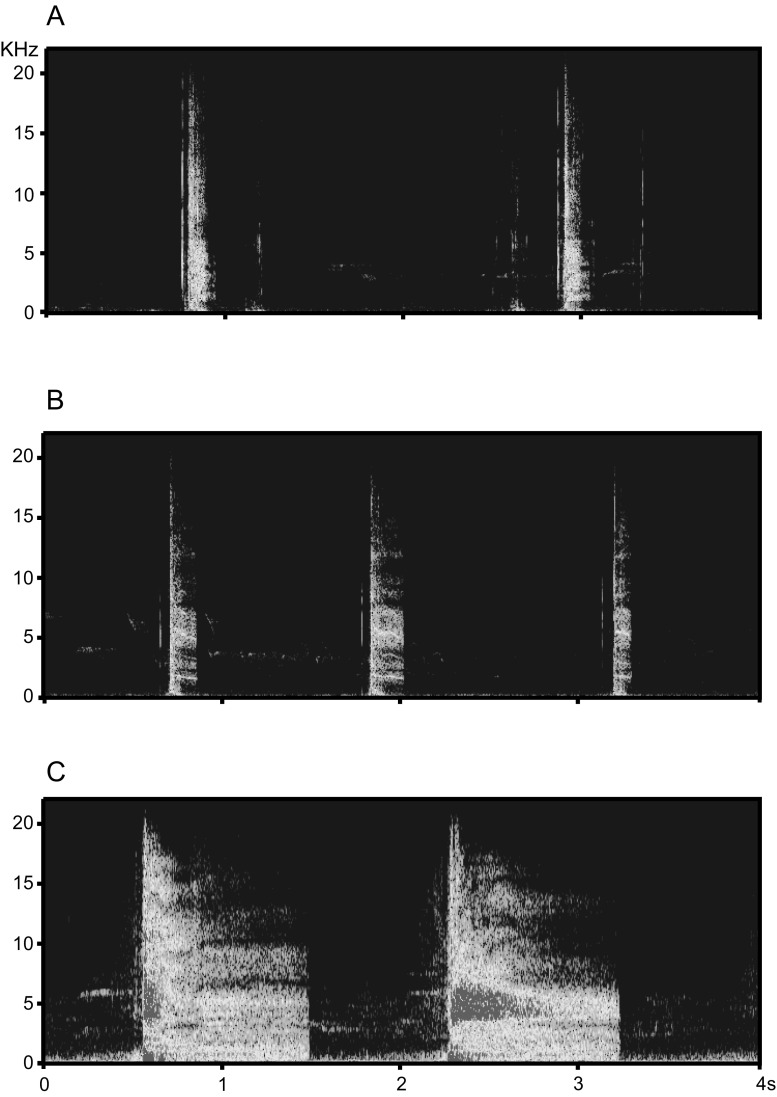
Sonograms (FFT length 512, sampling rate 44.1 kHz) of hissing calls made by Great Tit (a), Blue Tit (b), Marsh Tit (c), generated in SasLab Pro Avisoft, Germany
